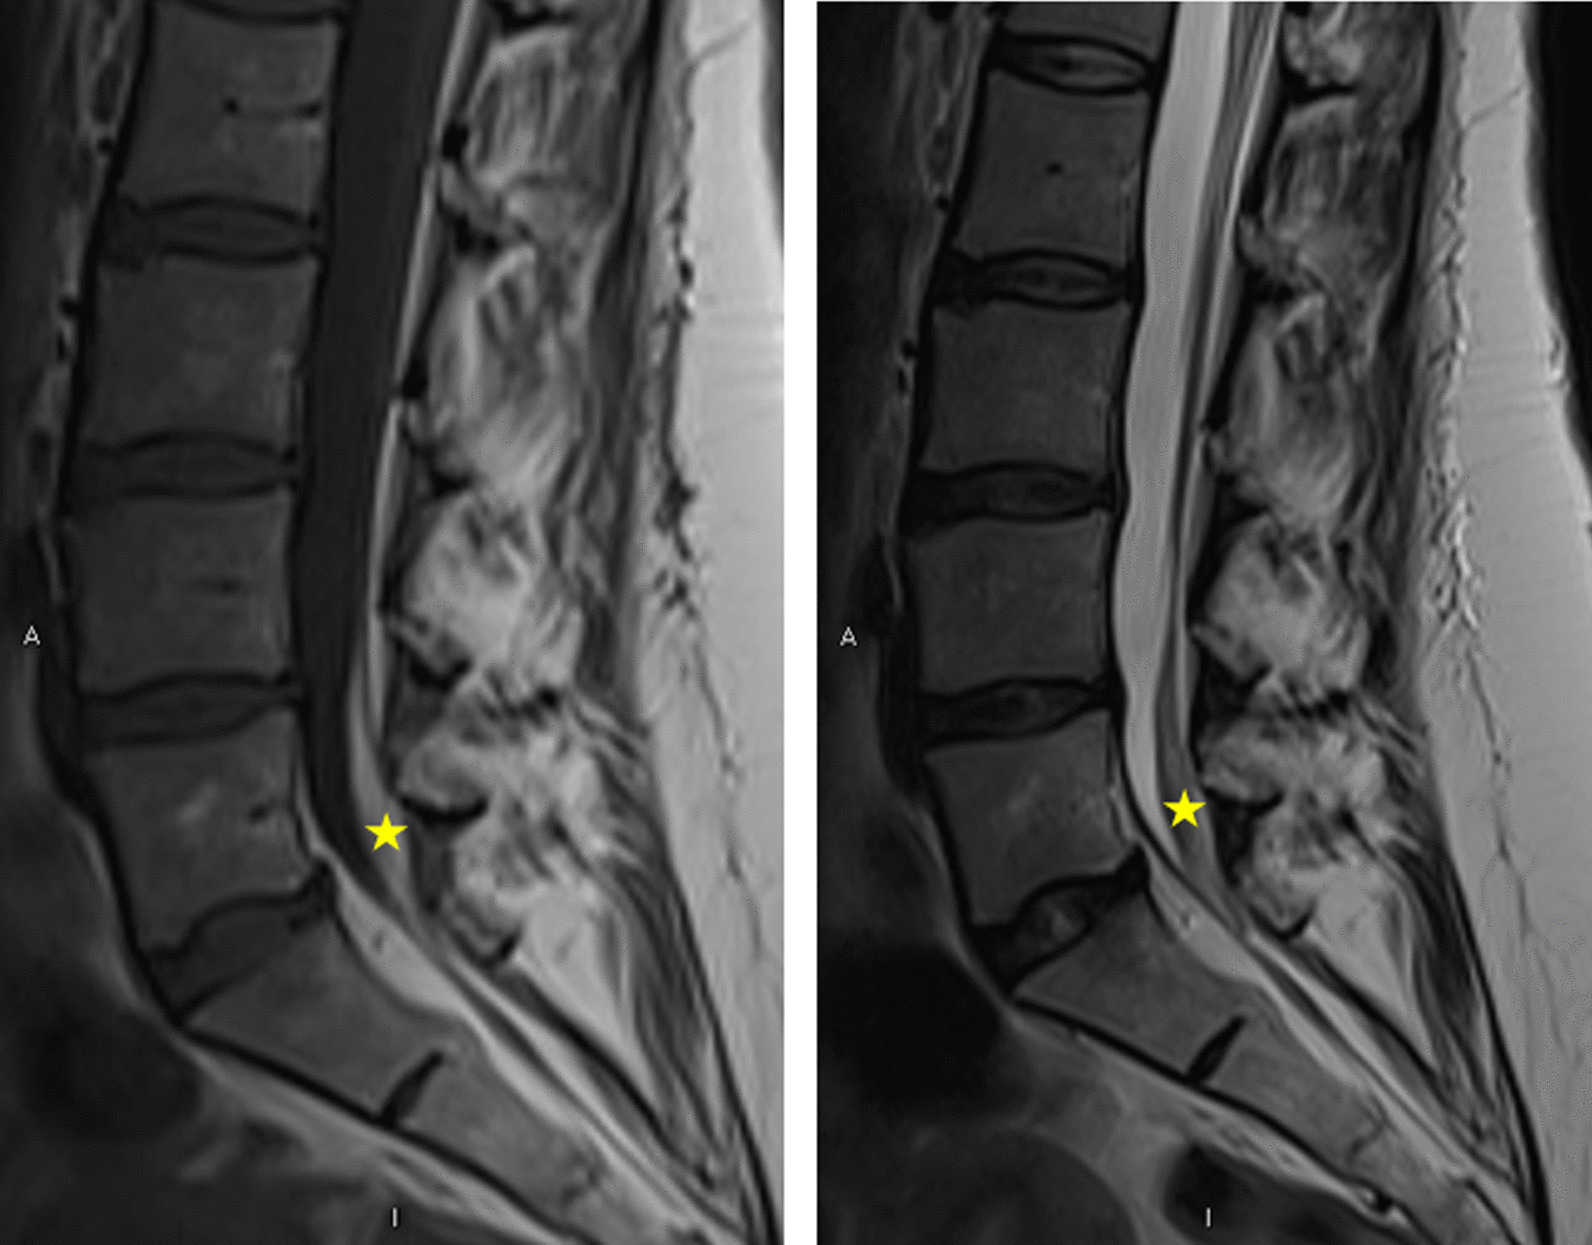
Initial images: Sagittal magnetic resonance imaging lumbar spine T1-weighted (left) and T2-weighted (right). There is a small volume T1 hyperintense intradural blood clot (asterisk) intermeshed with cauda equina nerve roots in posterior aspect of the thecal sac at L5 vertebral level. On the T2 weighted sequence, the clot appears relatively hypointense to the cerebrospinal fluid
